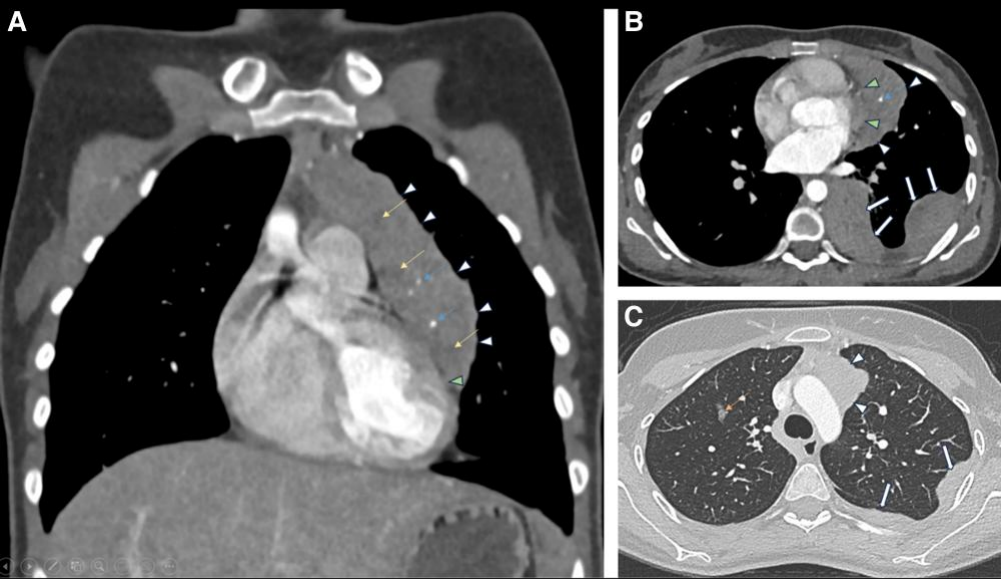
Selected images from contrast-enhanced chest CT scan demonstrated a large lobulated left anterior mediastinal mass (white arrowheads) with intrinsic coarse calcifications (blue arrows) and hypodense areas of necrosis (yellow arrows) in the soft tissue window coronal (A) and axial (B) reconstructions. There is a left pericardial invasion (green arrowheads). Multifocal left pleural nodules and masses (white solid arrows) representing solid metastases are also seen. Lung window axial reconstruction (C) reveals right lung upper lobe parenchymal ground glass nodules (orange arrow) in addition to the mediastinal mass (white arrowheads) and pleural deposits (white solid arrows). Features are consistent with invasive thymoma.
